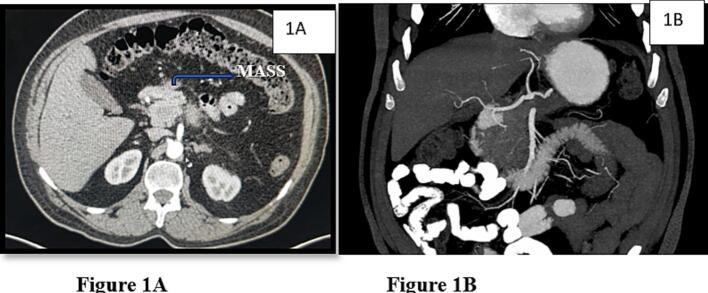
A. Axial contrast-enhanced CT showing a 4-cm hypodense mass in the body of the pancreas encasing the celiac axis. The lesion demonstrates loss of fat planes and circumferential contact with the celiac trunk, indicating locally advanced pancreatic ductal adenocarcinoma. B. Coronal CT image highlighting the tumor's relationship to the celiac axis and proximal splenic artery. The image emphasizes the loss of intervening soft tissue and the tumor's proximity to key vascular landmarks.
